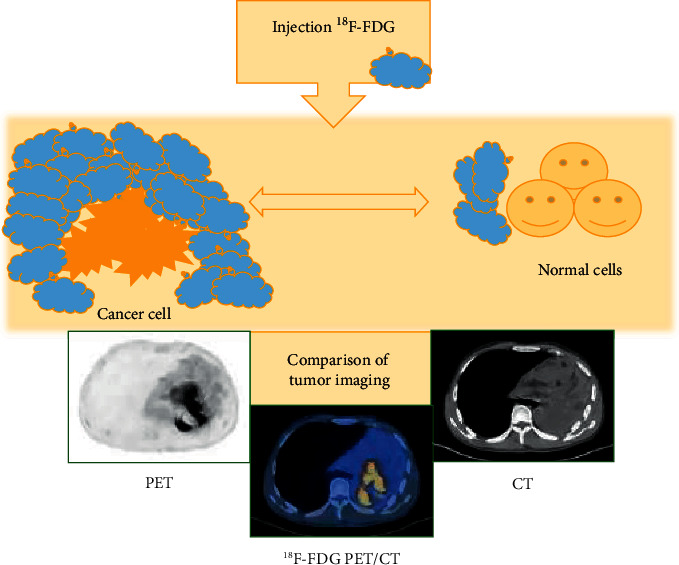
The imaging principle of ^18^F-FDG PET/CT.
